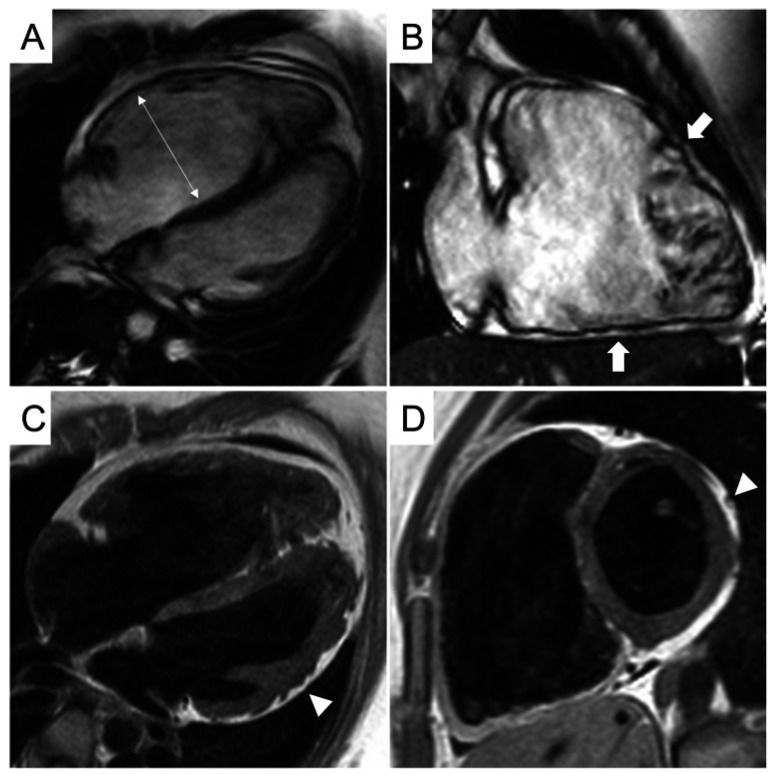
CMR frames of a patient with biventricular ACM. Morpho-functional abnormalities of the RV can be appreciated on a 4-chamber view (A) and right ventricular 2-chamber long-axis view (B) of cine images, evidencing RV dilatation (A, double-head arrow) and multiple sacculations of the inferior and RVOT regions (B, arrows). Structural alterations of the LV are showed in a 4-chamber view (C, arrowhead) and short-axis view (D, arrowhead) of T1-weighted images, where fibro-fatty infiltration of the infero-lateral LV walls becomes evident as a hyperintense signal with a typical bite-like pattern. ACM, arrhythmogenic cardiomyopathy; LV, left ventricle; RV, right ventricle; RVOT, right ventricle outflow tract. Reproduced from: Cipriani, A., et al. (2023) [39]. Licensed under CC BY 4.0 (https://creativecommons.org/licenses/by/4.0/ accessed on 15 December 2025).
